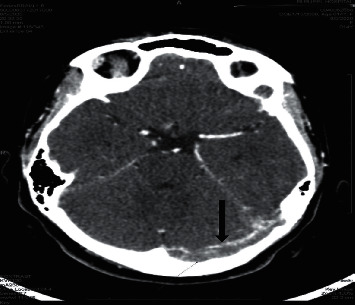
Plain CT scan axial cut shows relative density along the left transverse sinus (arrow).
